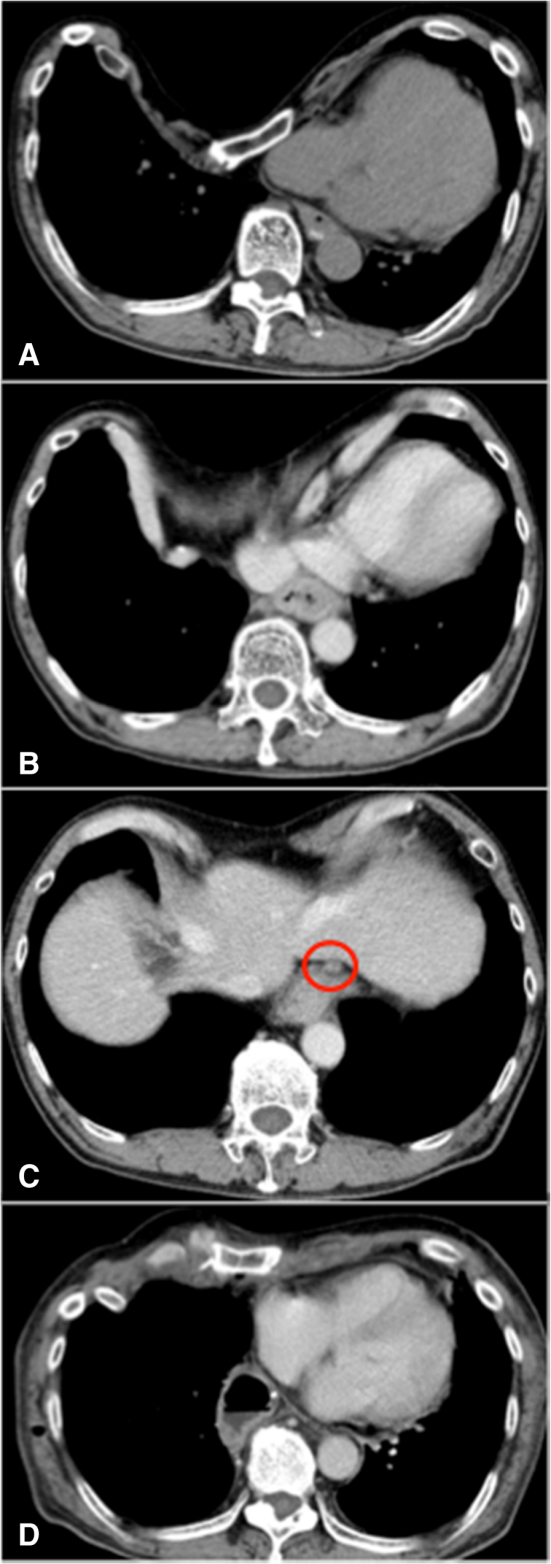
Chest computed tomography scan a Funnel chest (Haller index, 9.9).b Thickening of the esophagus.c The swollen lymph node (red circle).d After funnel chest surgery (Nuss method).
